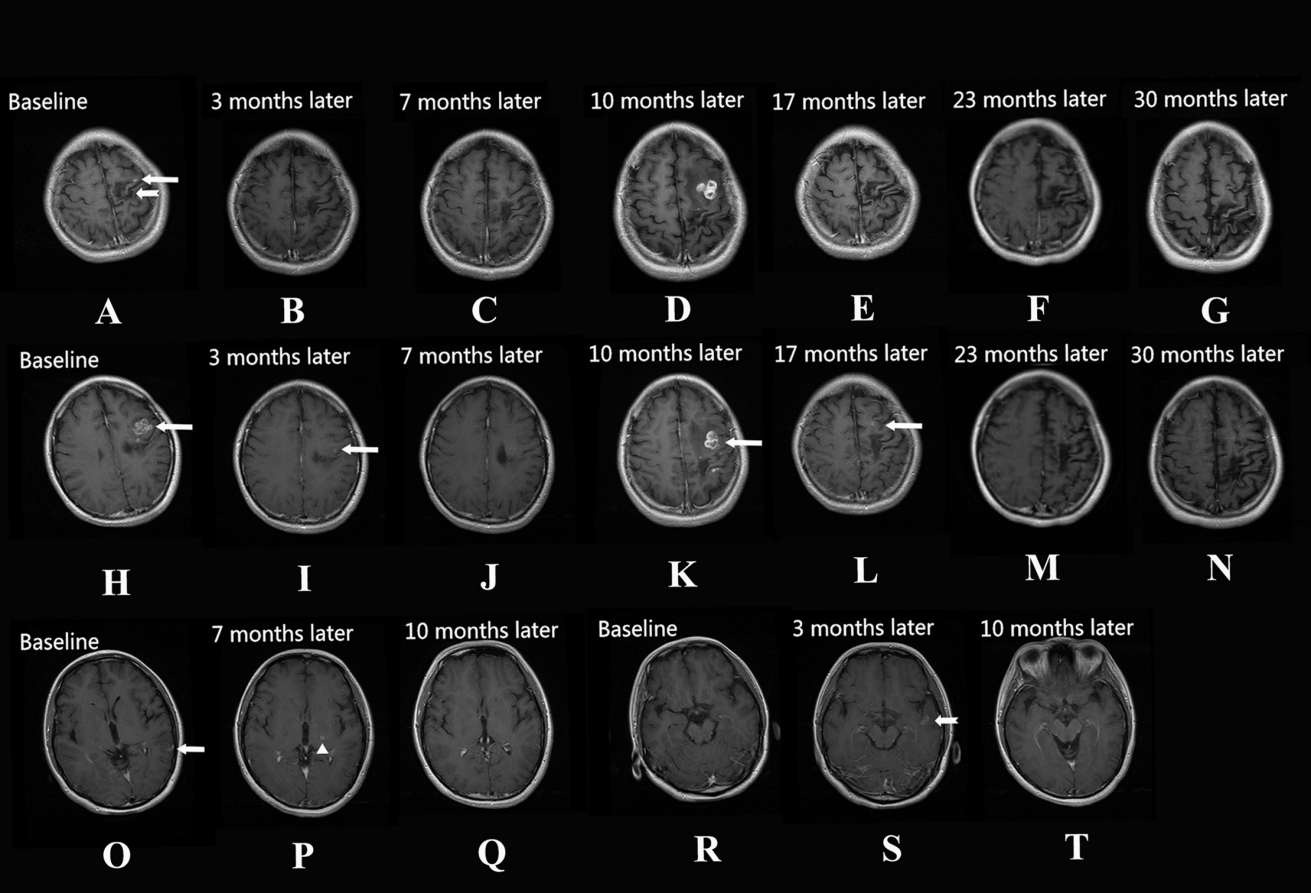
Axial images of a 22-year-old female patient with a 15-year history of seizures. Case 8. (A) (H). An axial T1 FLAIR image shows that there are aggregated ring-like, serpiginous and irregular enhanced lesions in the left frontal and parietal lobes (white arrows) at baseline MRI. White matter degeneration and cortical atrophy were found in the left frontal lobe (white open arrow) at baseline MRI. (B-G) (I-N). An axial T1 FLAIR image shows that after 2 courses of high-dose praziquantel therapy (7 months later), the left frontal lobe motile lesions disappeared. However, the initial aggregated ring-like and serpiginous enhanced lesions in left frontal lobe (white arrows) and parietal lobes reoccurred 10 months later. After 4 courses of therapy (17 months later), the extent of the left frontal lobe lesions (white arrow) are reduced significantly, and the left parietal lobe lesions disappeared. After 5 courses of therapy (23 months later) and follow-ups for 30 months, the left frontal lobe lesions disappeared completely, and white matter degeneration and cortical atrophy occurred in the corresponding area. (O-T) An axial FLAIR image shows that migrated lesions occurred in the left temporal lobe (3 months later) (white arrow and white open arrow) and the basal ganglia (7 months later) (white arrowhead) in follow-up MRI. After 2 courses of therapy (10 months later), the migrated lesions disappeared completely, and no long-standing lesions occurred.
